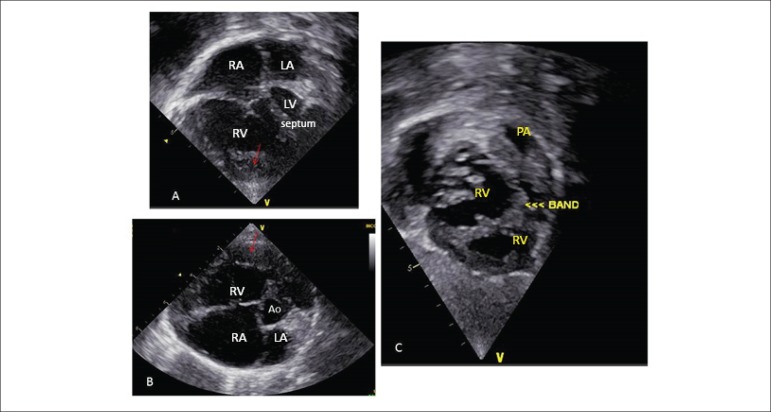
Echocardiogram: 4-chamber (A) and short-axis (B) views showing
